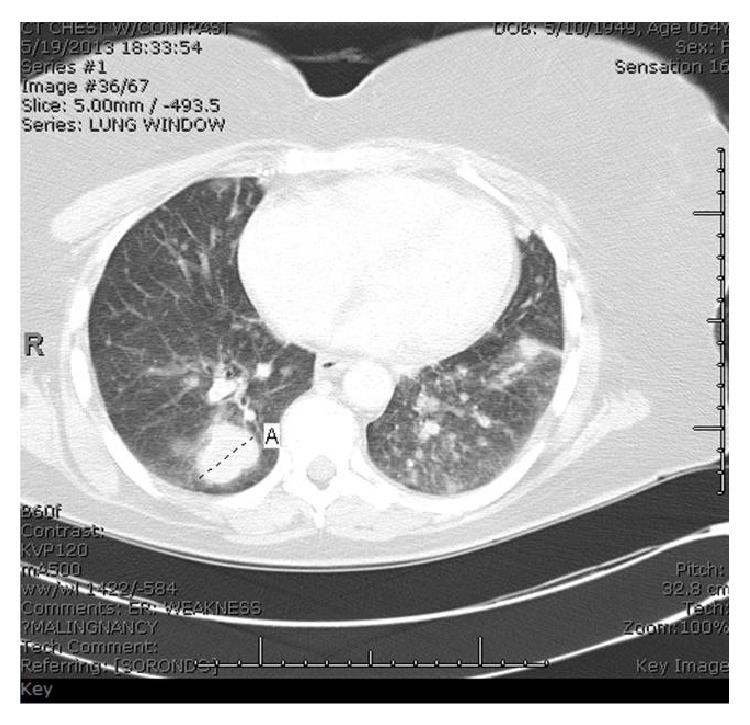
CT of the chest showing bilateral pulmonary nodules.
